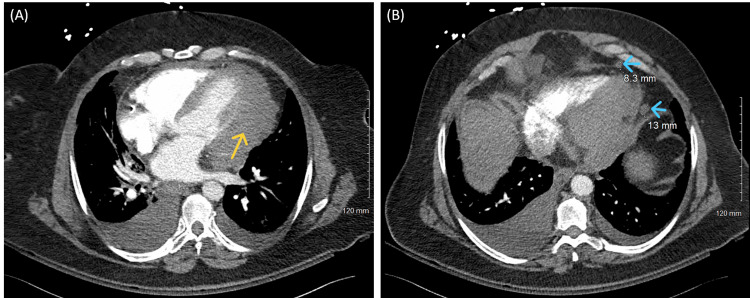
CT angiogram images demonstrating mild lobulated soft tissue density (yellow arrow) contiguous with the left ventricular myocardial wall extending to the left anterior mediastinum (A). Also seen are prominent pericardiac lymph nodes (blue arrows) measuring 8.3 mm and 13 mm (B).
